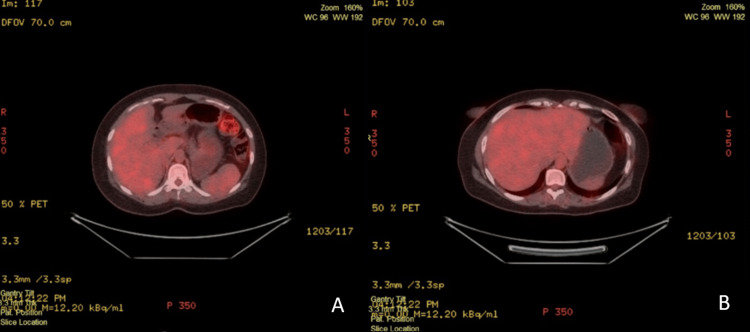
Follow-up FDG PET/CT showing complete metabolic response after chemo-immunotherapy. (A and B) Follow-up FDG PET/CT scan after three cycles of chemo-immunotherapy showing complete metabolic resolution of previously noted hepatic and nodal metastases.
